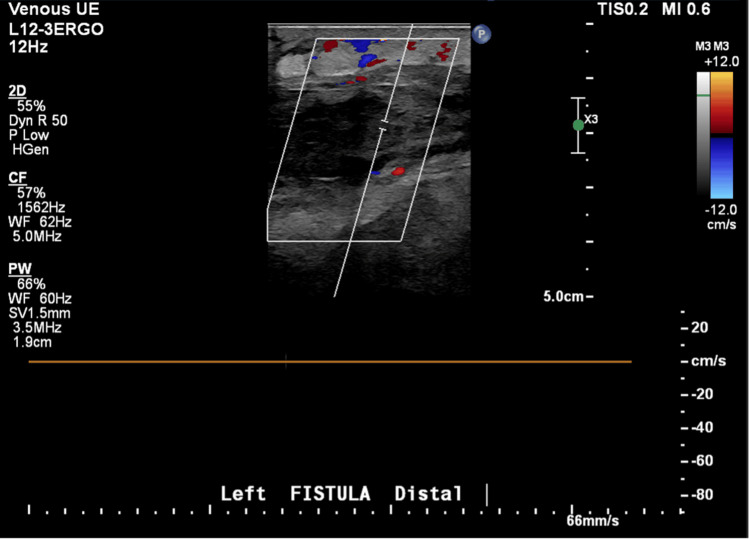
Left upper extremity venous duplex ultrasound.
